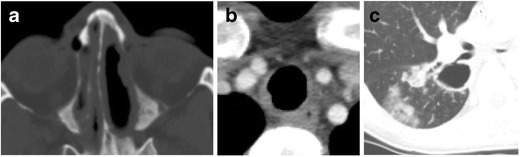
Twenty-nine-year-old woman with history of granulomatosis with polyangiitis. Axial CT images demonstrate a nodular thickening of the mucosa within the paranasal sinus, b eccentric soft tissue thickening of the tracheal wall, and c cavitary pulmonary nodules
